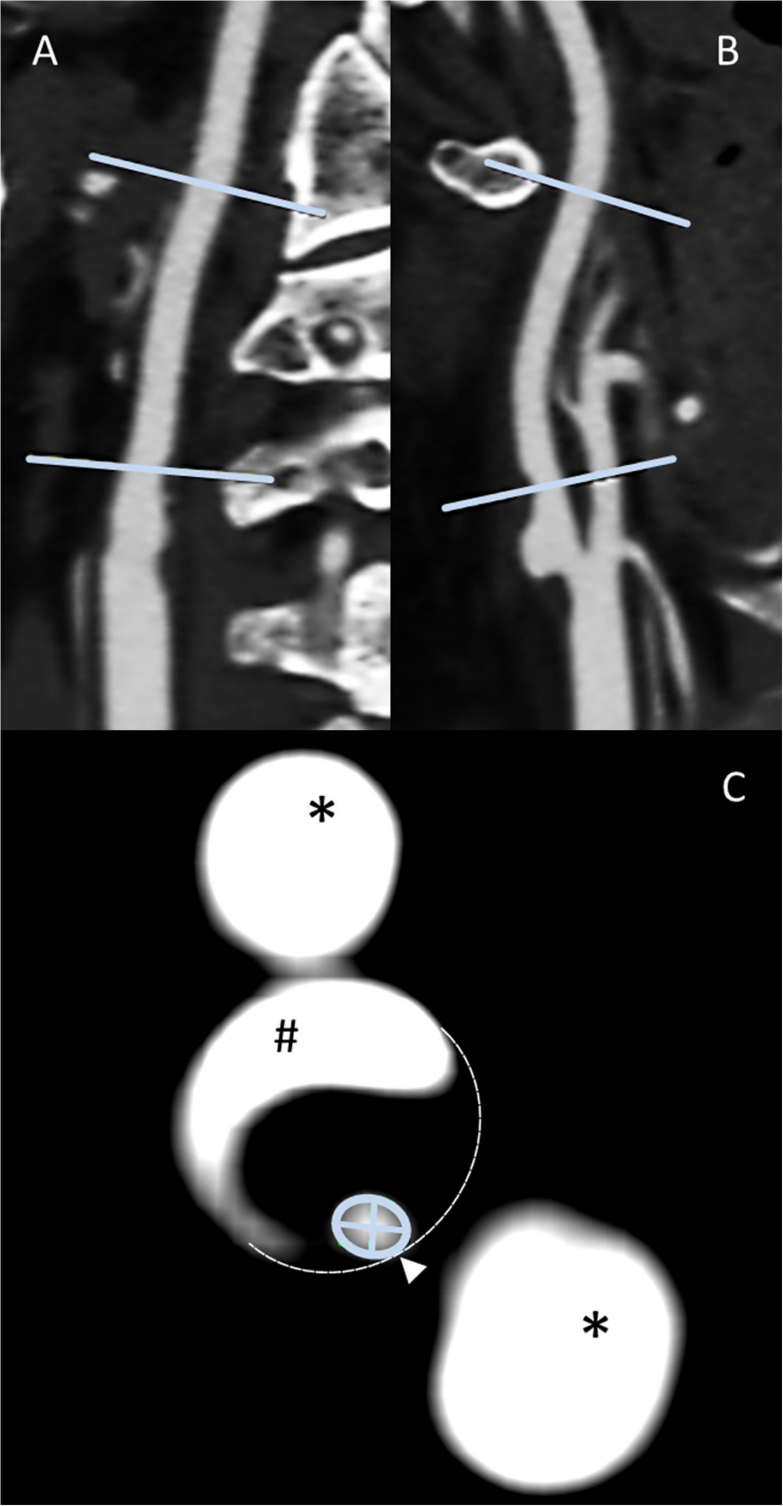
Methodology panel, MPR-based ICA stenosis diameter measurements: sagittal (A) and coronal (B) multiplanar reconstructions allow the identification of both the non-stenotic distal diameter [NASCET (C)] and the stenosis region. After the adjustment of either the coronal or sagittal plane for the optimal perception of the stenotic vessel configuration along the stenotic segment principal orientation, the subsequent perpendicular axial reconstruction of excellent spatial CTA resolution allows the outstanding distinction of vascular pathology (C), that is, calcified plaque (#), non-contrasted soft plaque (area enclosed by dashed line), and residual ICA lumen (encircled by green line). This plane is used for locating the smallest diameter [NASCET (A)]. Finally, the minimum stenotic diameter can be measured defined as the smaller of the two measurable diameters. External carotid artery branches (*) appear as homogenously contrasted additional lumina.
